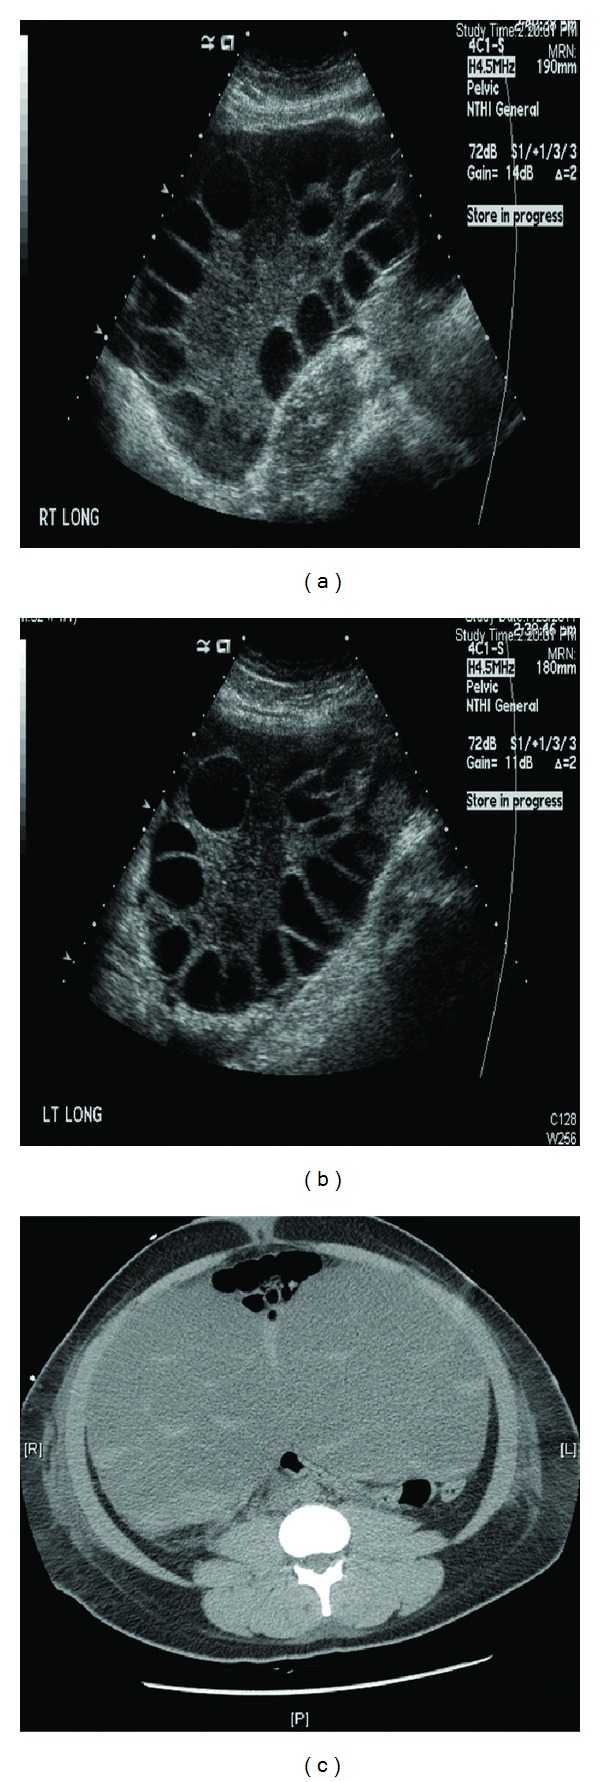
Ultrasound images showing enlarged right and left ovaries with multiple peripherally enlarged follicles. (a) Right ovary measuring 18 × 14 × 11 cm^3^, (b) left ovary measuring 17 × 12 × 12 cm^3^, and (c) computed tomography of the abdomen and pelvis showing bilateral enlarged multilocular and cystic ovaries.
